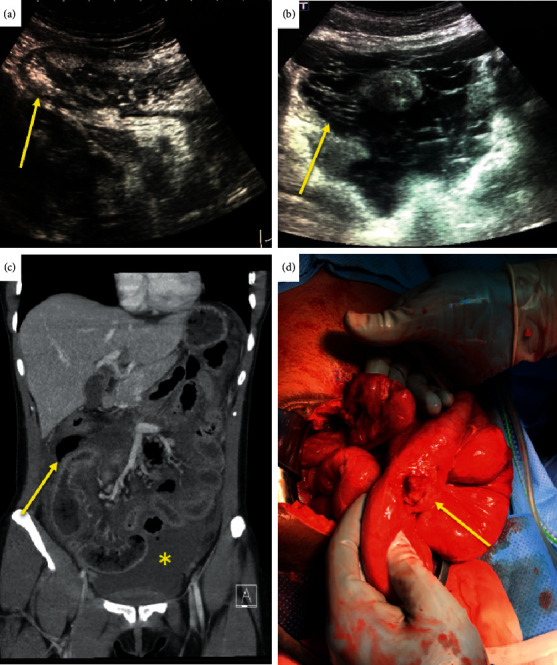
Ultrasound imaging reveals submucosal ileal wall thickening (a) (arrow) and free and loculated ascites (b) (arrow). Subsequent contrast-enhanced abdominal computed tomography showing ascites (asterix) and free air (arrow) in the peritoneal cavity in close relation to affected terminal ileum wall (c). Laparotomy showed many adhesions and focal omental thickening adhering to a thickened distal ileum loop cavity (d) (arrow).
